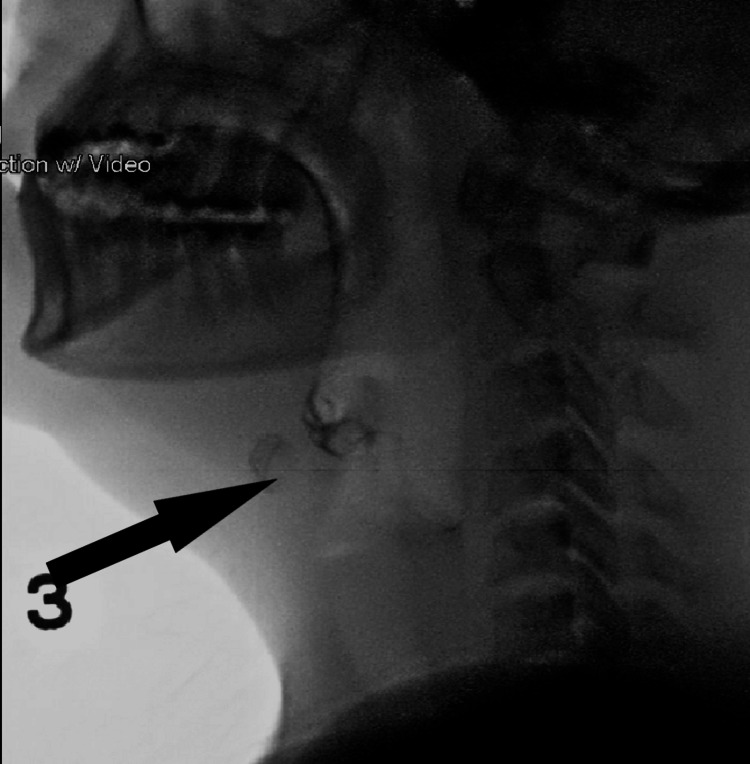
X-ray video fluoroscopy image with an arrow pointing toward the pooling of contrast in the valleculae
